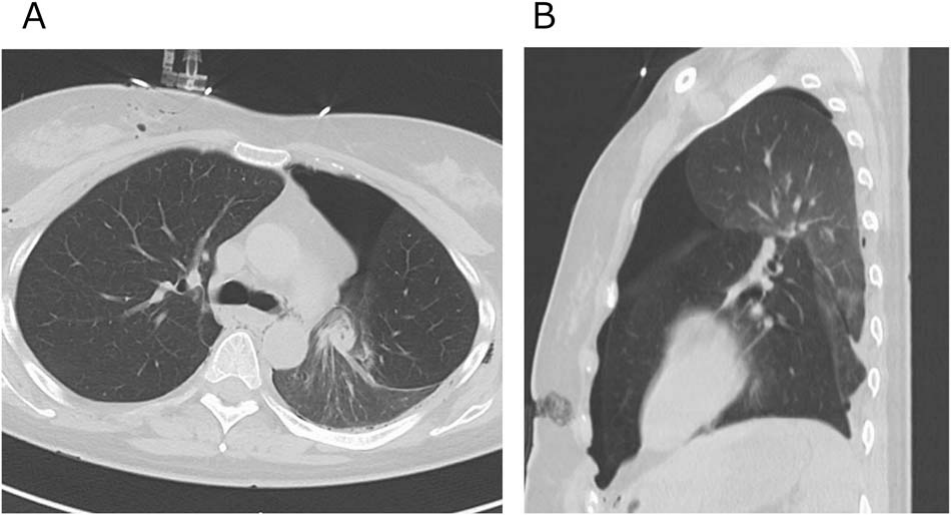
Axial (A) and sagittal (B) computed tomography images few hours after initial emergency operation.
